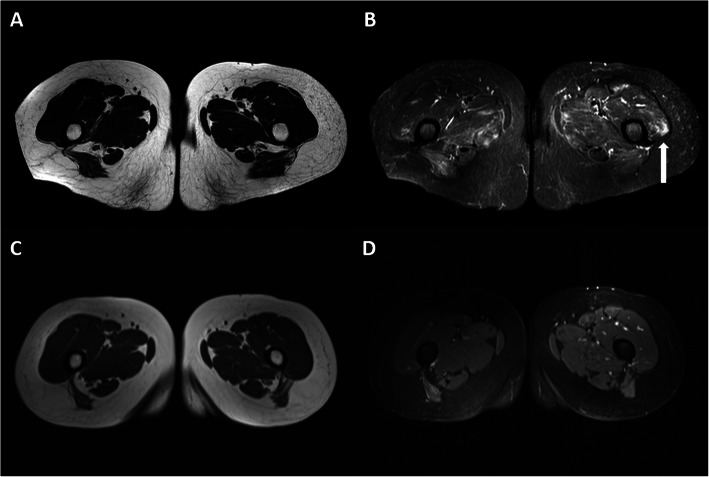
MRI thighs pre- and post-rituximab therapy. Axial a T1-weighted FSE and b STIR images of the upper thighs in May 2018 showing patchy oedema within the bilateral vasti, adductors and hamstrings. Signal abnormality in the left vastus lateralis is most conspicuous (white arrow). Subsequent axial c T1-weighted FSE and d STIR images of the same region in March 2019 demonstrate complete resolution of oedema. Muscle bulk remains normal on both studies with no fatty infiltration
